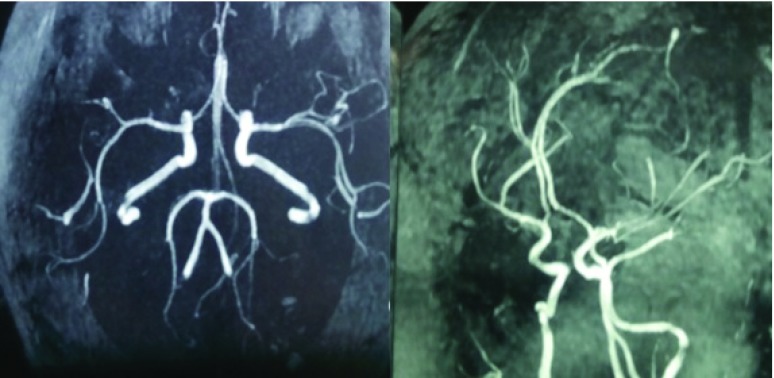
MRI angiography showing absence of any vascular blush or major arterial feeder to the lesions.
